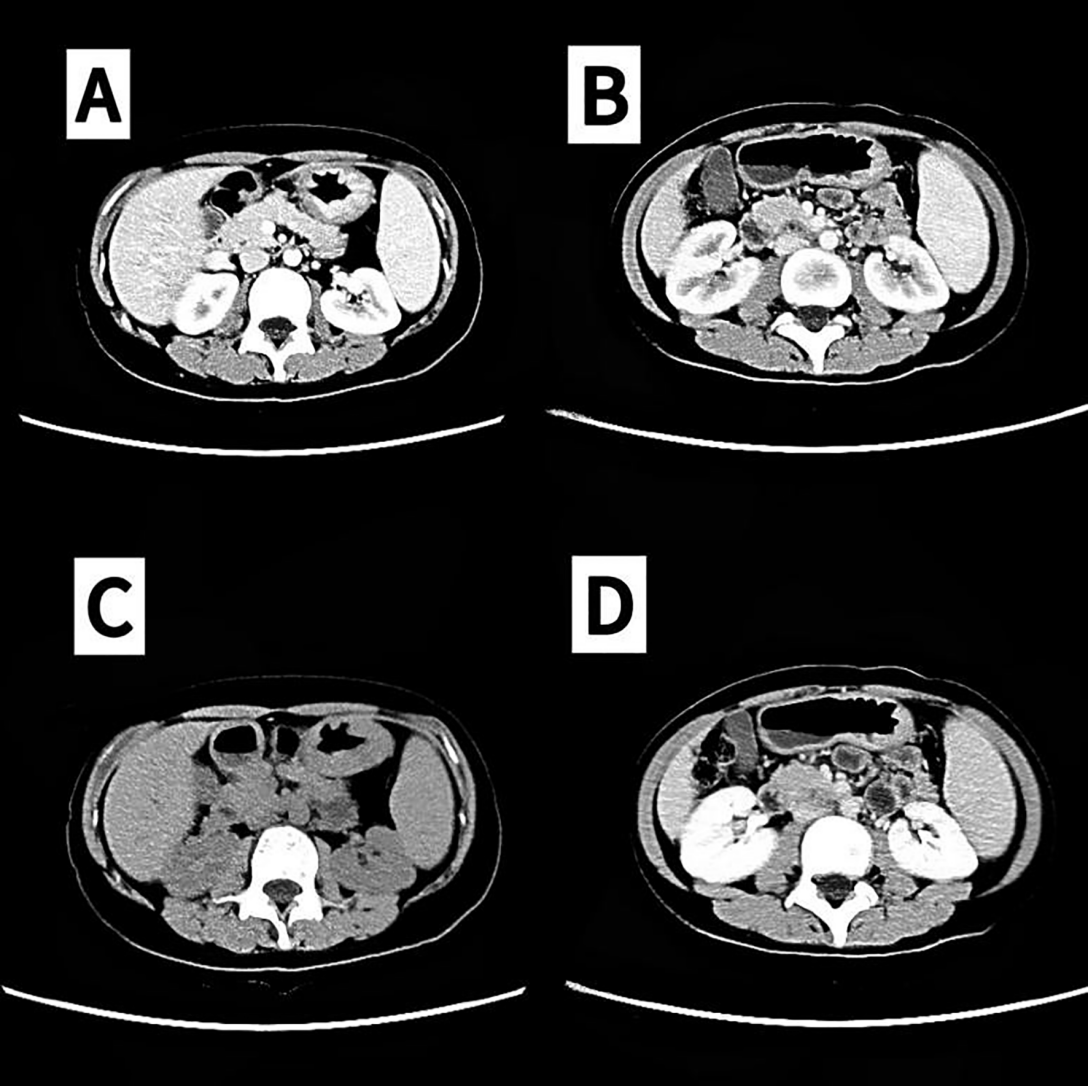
Partial remission (PR) confirmed by computed tomography (CT). (A, C) 2024-01-25. (B, D) In 2024-03-28, partial remission (PR) was assessed via CT (lymph nodes in the hepatogastric hiatus, splenogastric hiatus, and mesenteric root section were reduced and minimized from previous levels).
